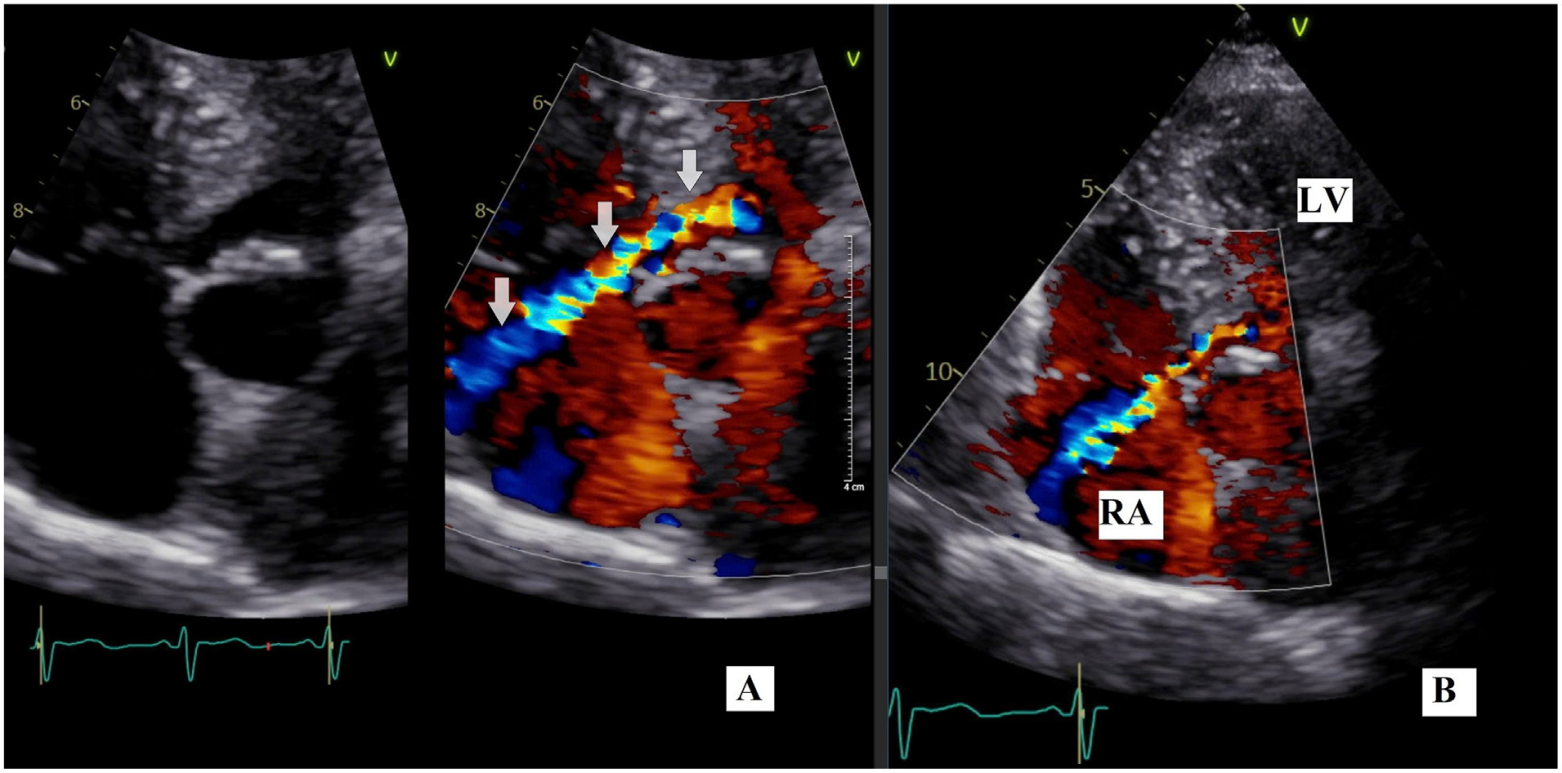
TTE in the second patient. (A-B) 2D and color Doppler views demonstrate a small left-to-right shunt from the left ventricle to the right atrium (white arrow).
